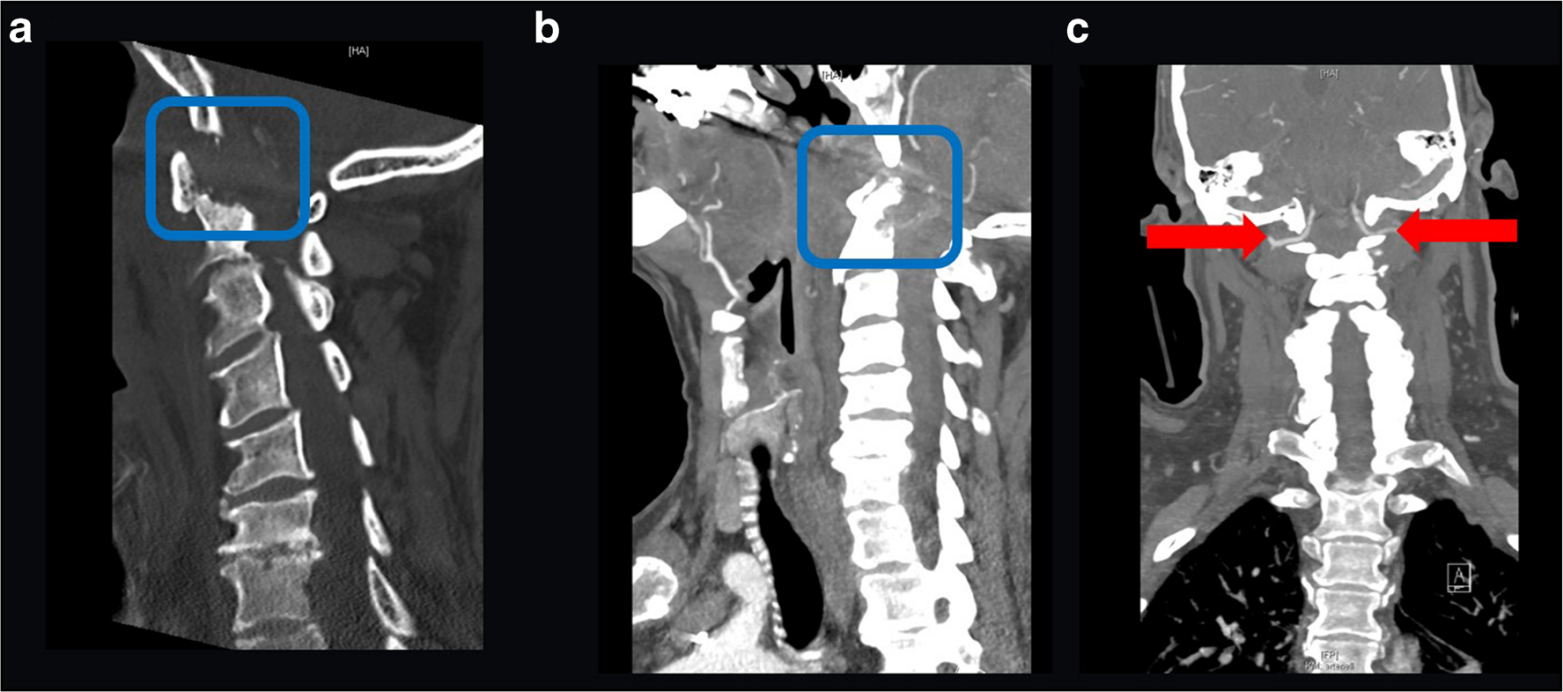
Preoperative CT and CTA of cervical spine: a sagittal CT (bone window) revealed an osteolytic lesion of the OP (blue frame); b sagittal CT (soft tissue window) showed compression of spinal cord (blue frame); c coronal CT revealed “normal” variant of V3 segment of VA (VA runs over the posterior arch of C1) (red arrows)
